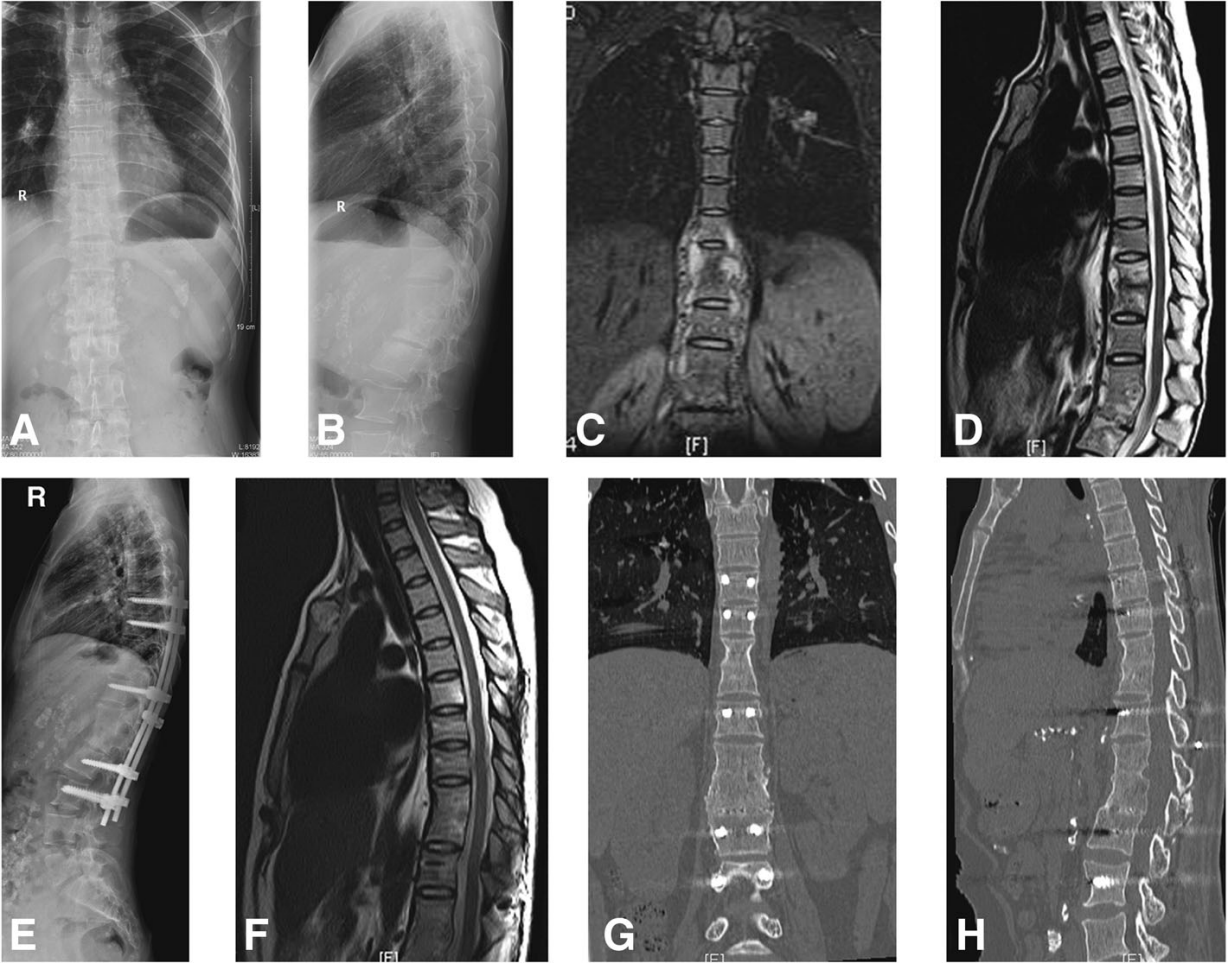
Preoperative radiography (a, b) and MRI (c, d) of a 23-year-old female patient with tuberculosis at T8–L3 with extensive paravertebral abscesses. Radiography (e), MRI (f), and CT (g and h) at the final follow-up showed definitive interbody bone fusion was achieved at T9–10 and T12–L1. MRI: magnetic resonance image; CT: computed tomography scan
